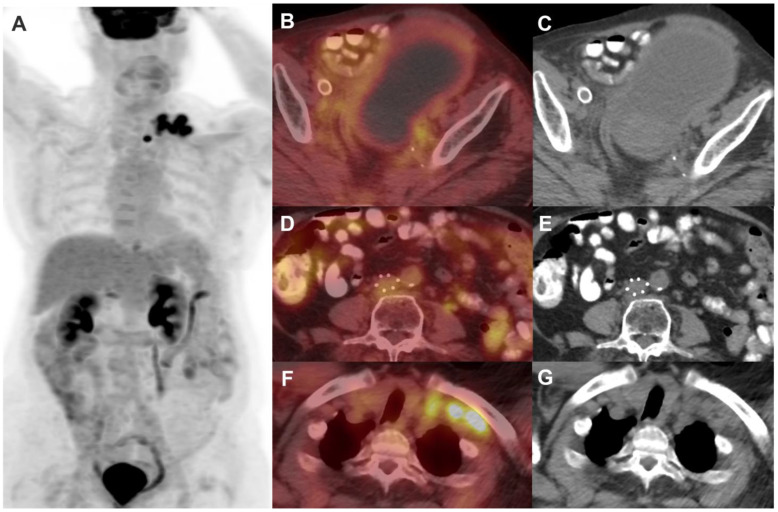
The same patient shown in Figure 4 underwent post-treatment follow-up FDG PET/CT 3 months after concurrent chemotherapy and external beam radiation to the pelvis and retroperitoneum. Maximum Intensity Projection image of FDG PET/CT (A) demonstrates a cluster of metabolically active left supraclavicular lymph nodes. There is resolution of FDG-avid primary cervical lesion, pelvic nodes (B,C), and retroperitoneal nodes (D,E). There is, however, an increase in the number and size of FDG-avid left supraclavicular lymph nodes (F,G), suggestive of disease progression outside the radiation treatment field.
